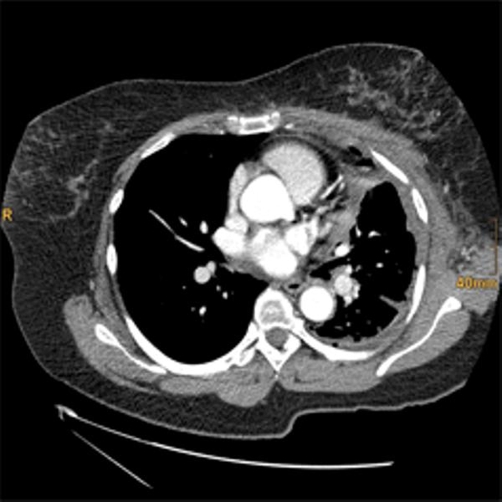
Chest computed tomography after resection of the tumor through a thoracotomy. This image shows volume reduction and fluid accumulation with no signs of relapse.
